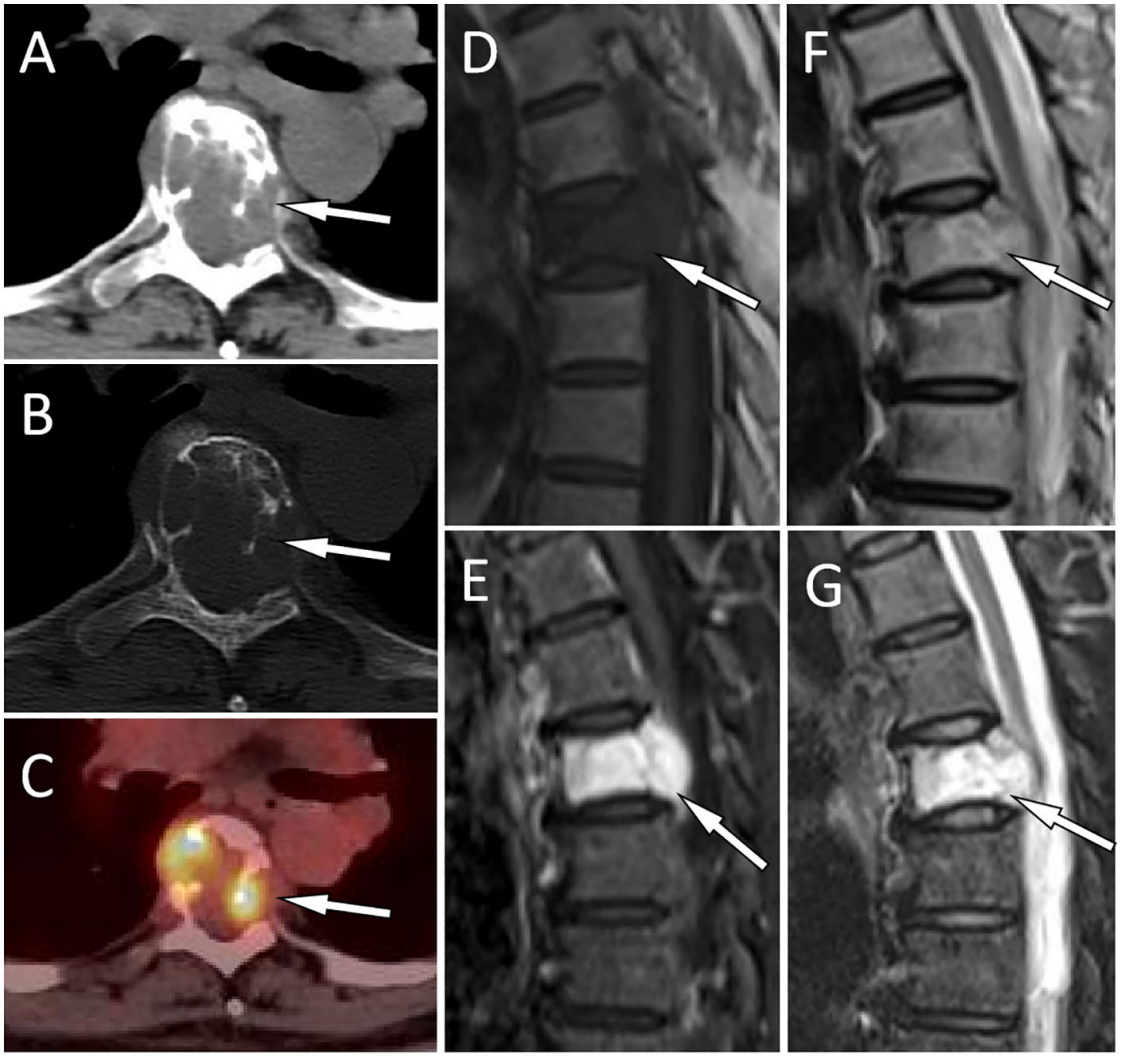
A 51-year-old woman with EH (case 1): The axial CT soft tissue window shows a soft tissue mass extending toward the periphery of the vertebral body and the left accessory as well as the spinal canal with T5 as the center [(A), arrow]. The CT bone window shows expansive osteolytic bone destruction in the T5 vertebral body and the left accessory, with residual bone trabeculae visible inside (arrow) and no sclerotic rim (B). Axial PET/CT fusion images showed moderate FDG uptake (arrow), with an SUVmax of 6.0 (C). Sagittal T1WI shows mild compression of the T5 vertebral body, and the lesion exhibits hypointensity changes [(D), arrow]. Contrast-enhanced T2WI shows significant enhancement of the lesion [(E), arrow]. T2WI sagittal imaging shows a slight hyperintensity of the lesion [(F), arrow]. Fat-suppression T2 sequence showing hyperintensity of the lesion [(G), arrow].
